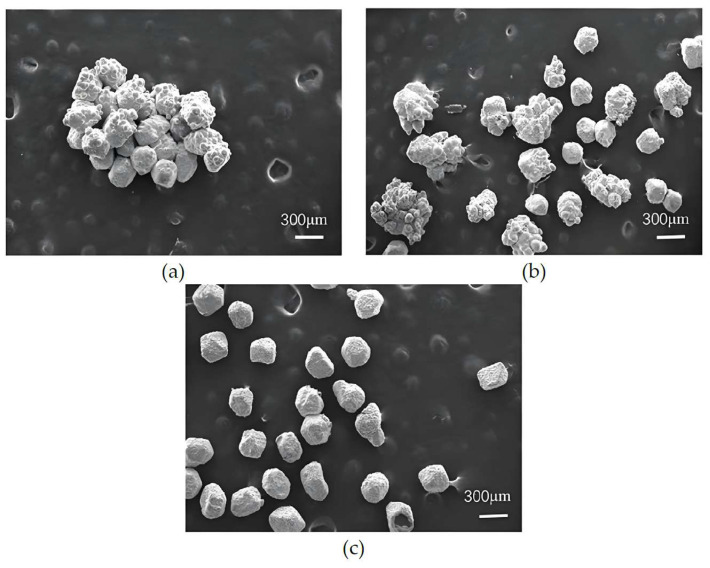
SEM images of nickel electroplating diamond under varying output power levels of ultrasonic oscillator: (a) 200 W; (b) 500 W; (c) 900 W.
